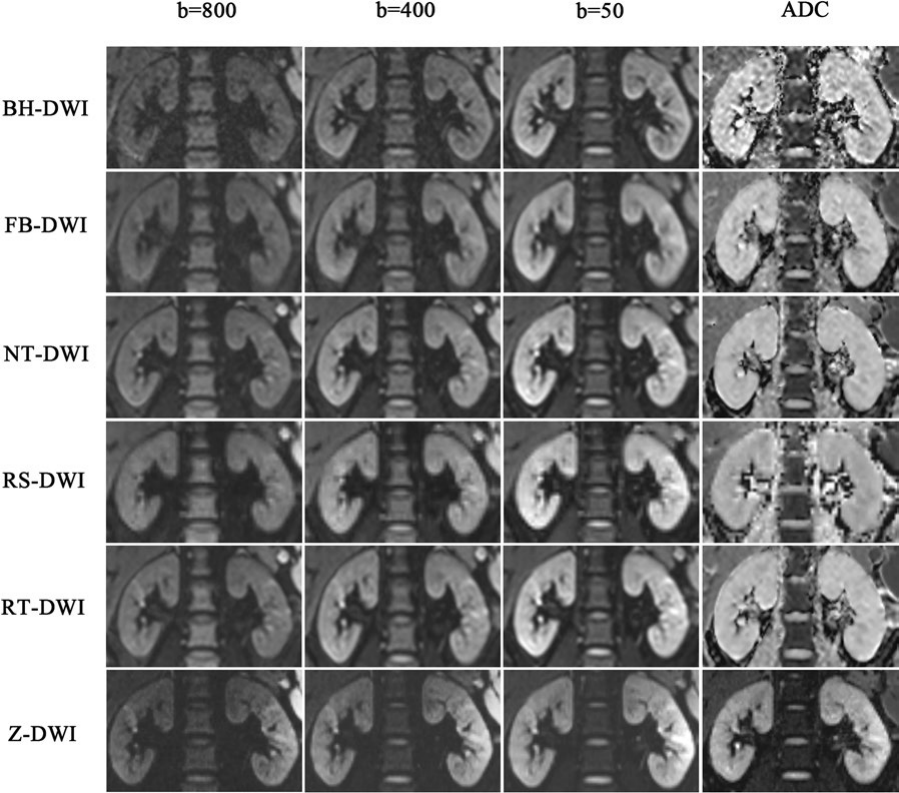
Comparisons of image quality of BH-DWI, FB-DWI, NT-DWI, RT-DWI, RS-DWI and Z-DWI. Diffusion-weighted trace images at three different b-values (800, 400, 50 s/mm^2^) with the corresponding ADC maps (right) are arrayed. Z-DWI had a better image quality than BH-DWI and FB-DWI in clarity of the renal cortex and medulla (K4) (ADC map) (all *P* < 0.05) and RS-DWI in sharpness of boundaries (K1), clarity of the renal cortex and medulla (K4) and overall image quality(K5) (all *P* < 0.05). Z- DWI was slightly superior to RT- DWI and NT-DWI in sharpness of boundaries (K1, ADC map); however, the difference in image quality between the three was not significant (all *P* > 0.05).
